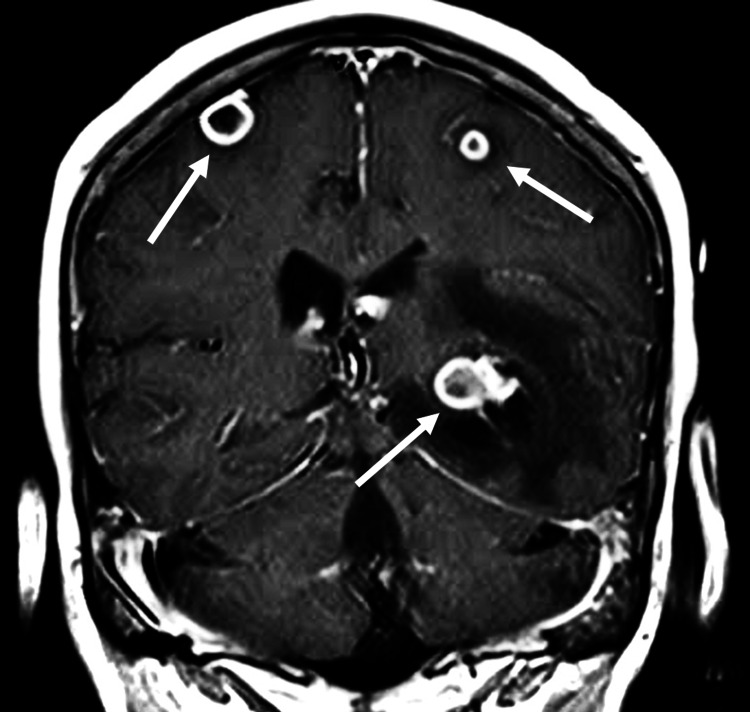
Cranial MRI coronal section Focal hypointense lesions in T1 with annular enhancement after contrast administration, involving both frontal and left temporal lobes
